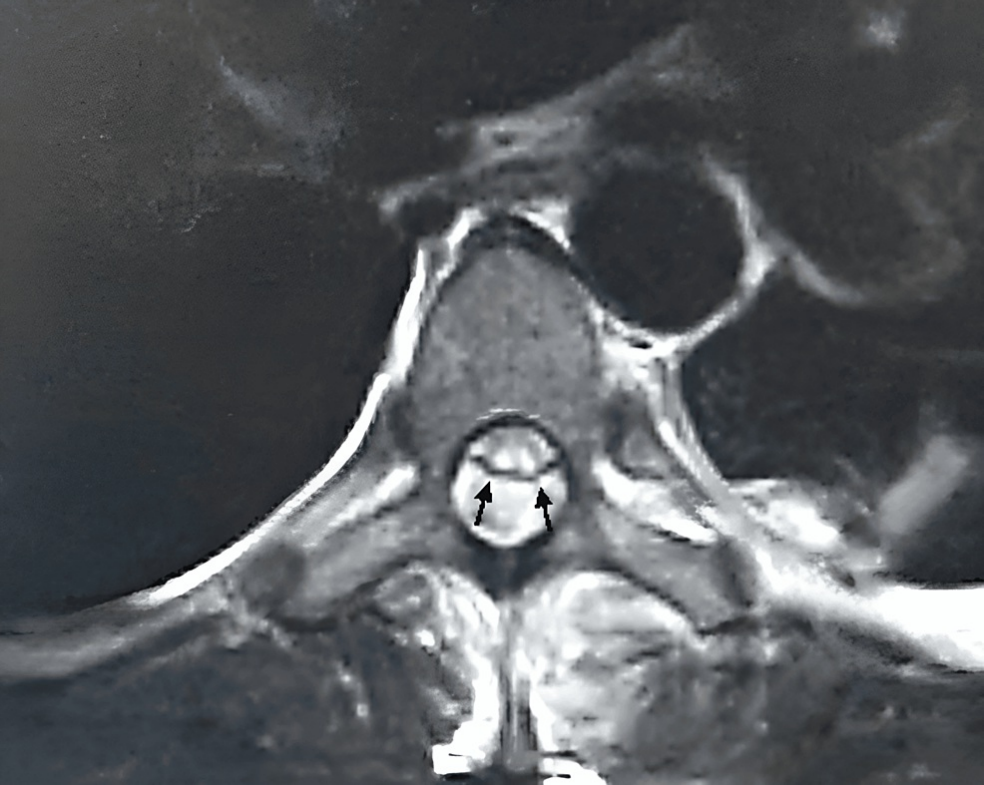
Axial T2-weighted MRI The image demonstrates an intradural-extradural spinal epidural abscess in the dorsal region at the T1 level, with ventral displacement of the spinal cord (arrows) MRI: magnetic resonance imaging
